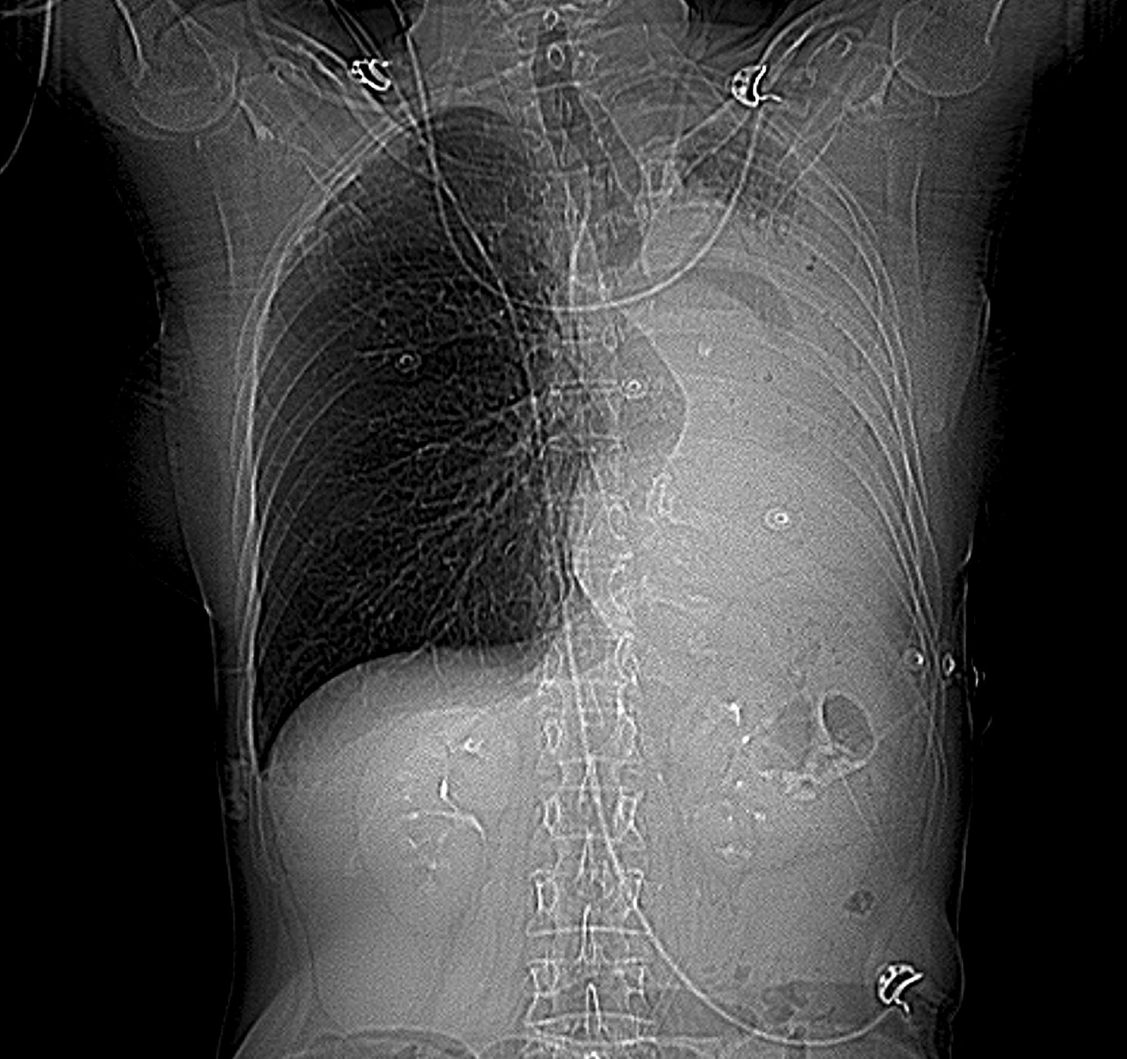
X-ray image prior to CT-scan with no air or pulmonary configuration on the left hemithorax
